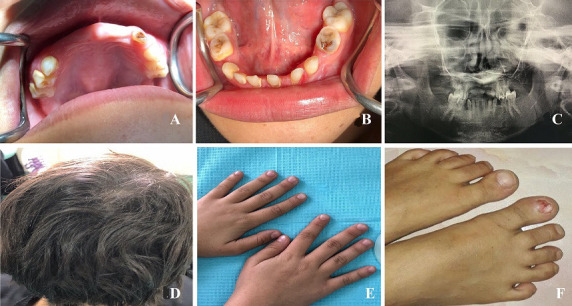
A) maxillary arch showing retained deciduous teeth, missing permanent teeth and palatal tori; B) mandibular arch showing retained deciduous teeth, missing permanent teeth; C) panoramic radiograph showing generalised absence of permanent tooth bud in both arch; D) thinning of hair; E) thin and brittle fingernails; F) toenails showing ridging and koilonychia
